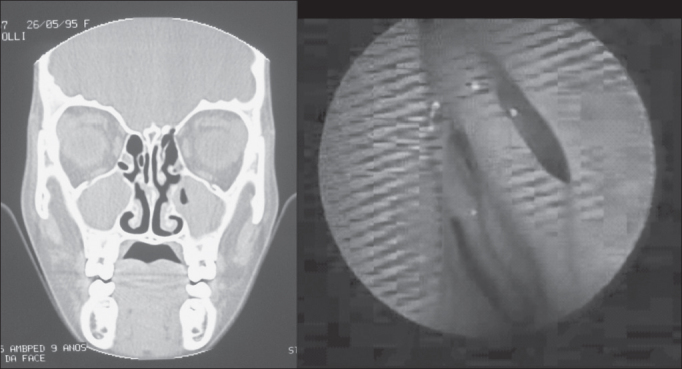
Example (case 5) of disagreement between the results of assessments. CT scan with positive diagnosis and nasofibroscopy with negative diagnosis of chronic rhinosinusitis.
